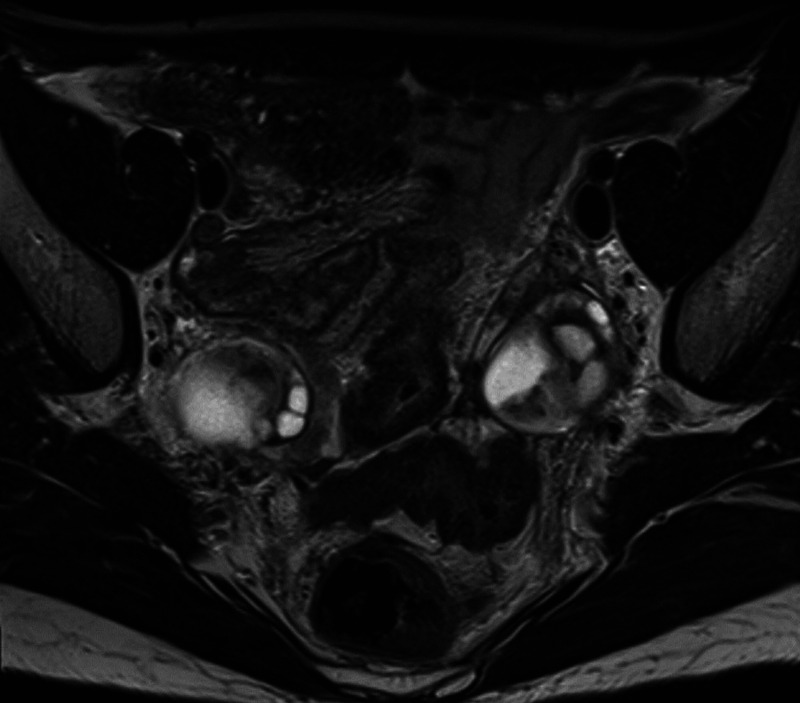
Axial T2 weighted MRI pelvis showing resolution of the pelvic abscesses and improvement in the bowel wall inflammatory change. The ovaries are cystic bilaterally.
